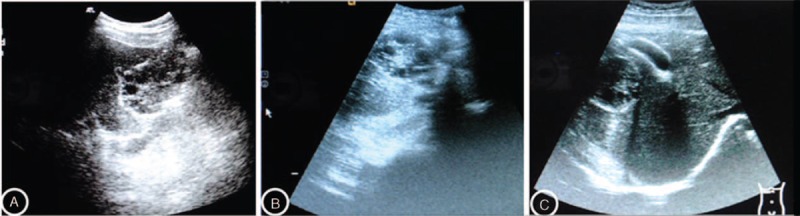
Ultrasound images of the CE lesions obtained from the baseline period (2A), 3 months (2B), and 6 months (2C) after administration of T-ABZ, respectively. The results indicated that after administration of T-ABZ was effective for treating CE. CE = cystic echinococcosis, T-ABZ = tablet-albendazole.
